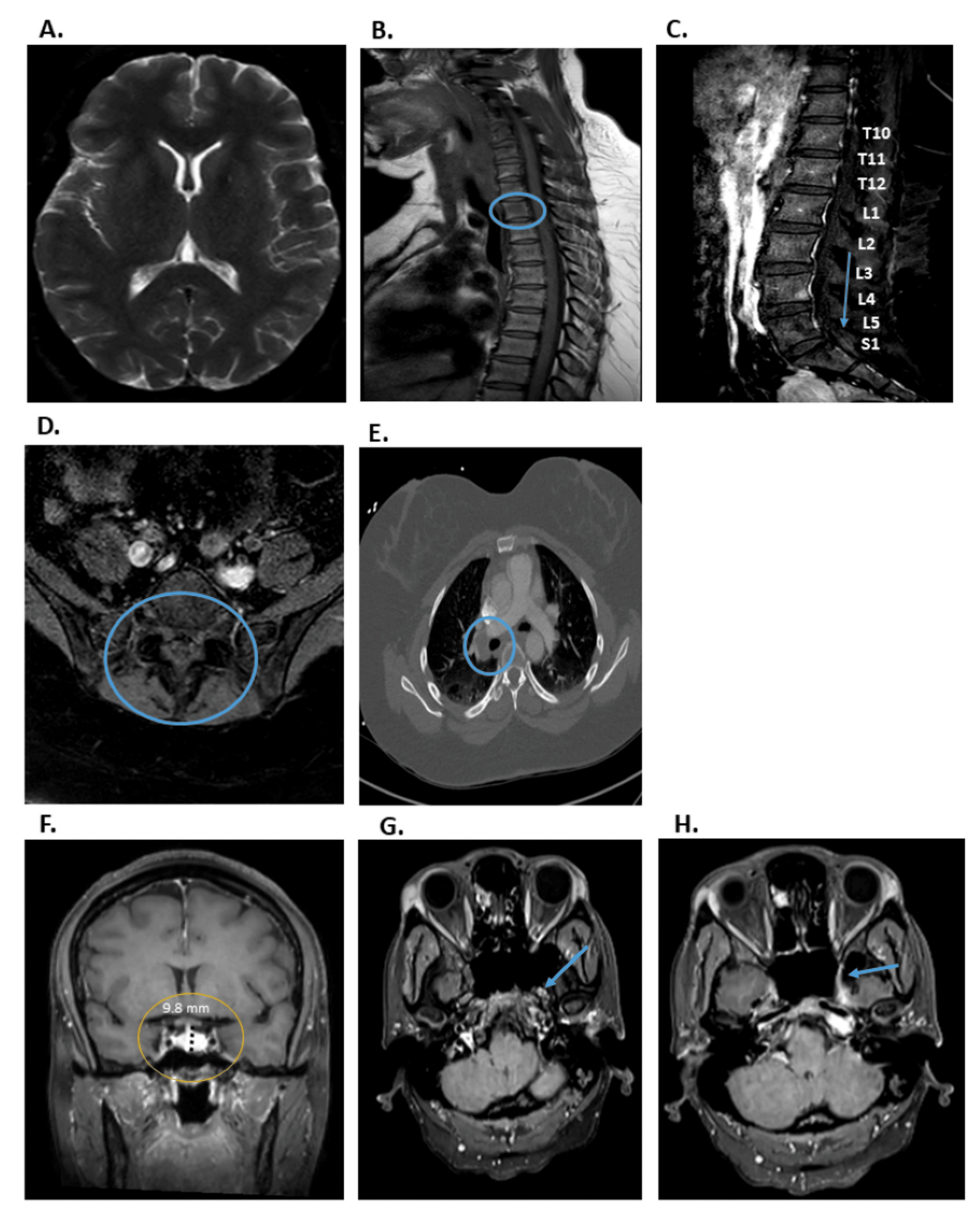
Representative MRI and CT scan images with and without contrast. (A) A representative picture of the initial brain MRI shows no intracranial lesions. (B) A representative picture of the thoracic spine MRI shows a hyper-intense lesion of the T2 vertebral body measuring approximately 9 mm. (C & D) Lumbar spine MRI showing leptomeningeal enhancement in the sacral canal at the level of S1. (E) Thoracic CT with and without contrast showing right hilar adenopathy. (F, G & H) Representative pictures of the repeat brain MRI show mild enlargement of the pituitary gland (9.8 mm) in the F panel and mild thickening and enhancement of the pituitary stalk in the G and H panels.
